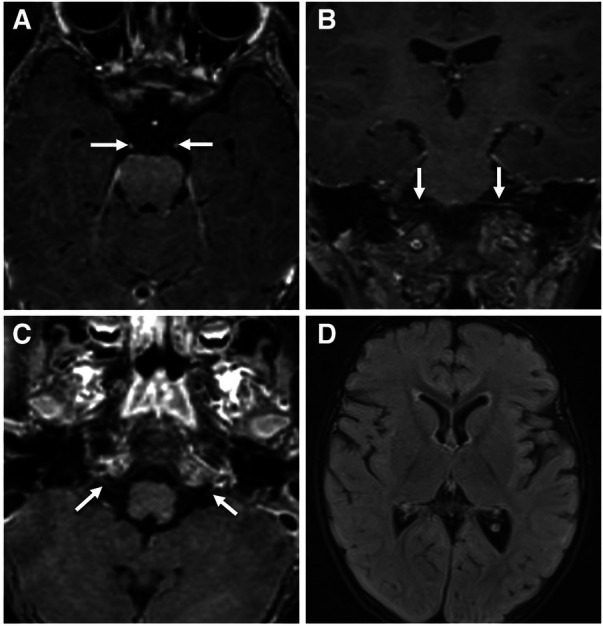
Contrast-enhanced brain MRI images acquired at 18-months of age demonstrate smooth bilateral enhancement of multiple cranial nerves, including the oculomotor nerves, cisternal and intracanalicular segments of the facial nerves and the glossopharyngeal nerves (white arrows in A–C). Axial FLAIR image (D) shows no signal abnormality in the brain parenchyma.
